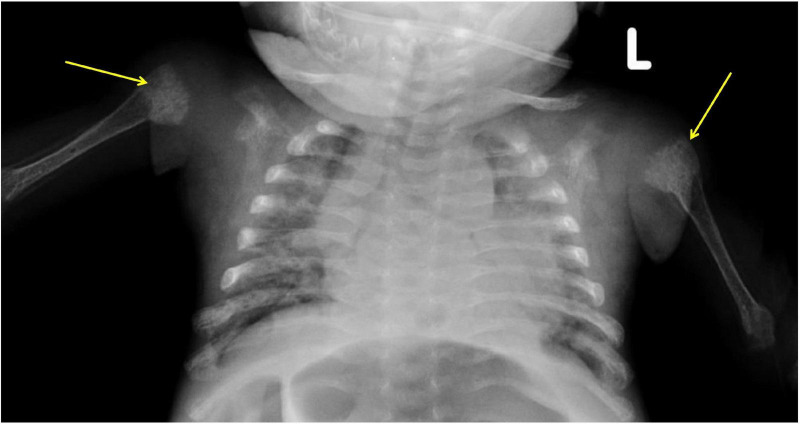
X-Ray chest: Both humeri were noted to be severely osteopenic with significant changes of rickets at proximal metaphyseal ends (arrows).
